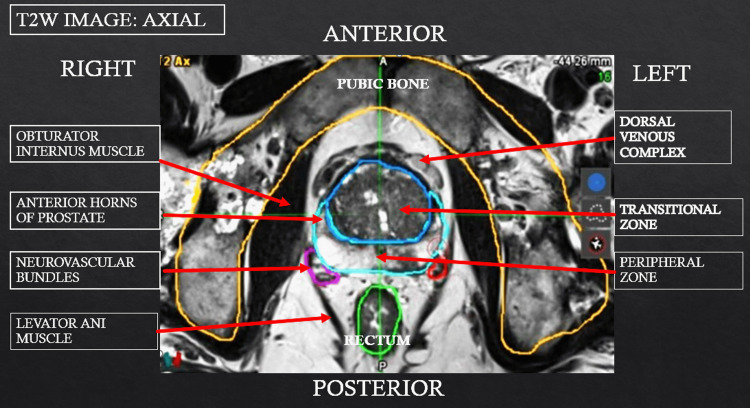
Advances in MRI software segmentation allows identification of key prostatic landmarks In this figure, an axial image depicts key structures related to primary and secondary continence control, including the levator ani muscles and obturator internus muscles.
